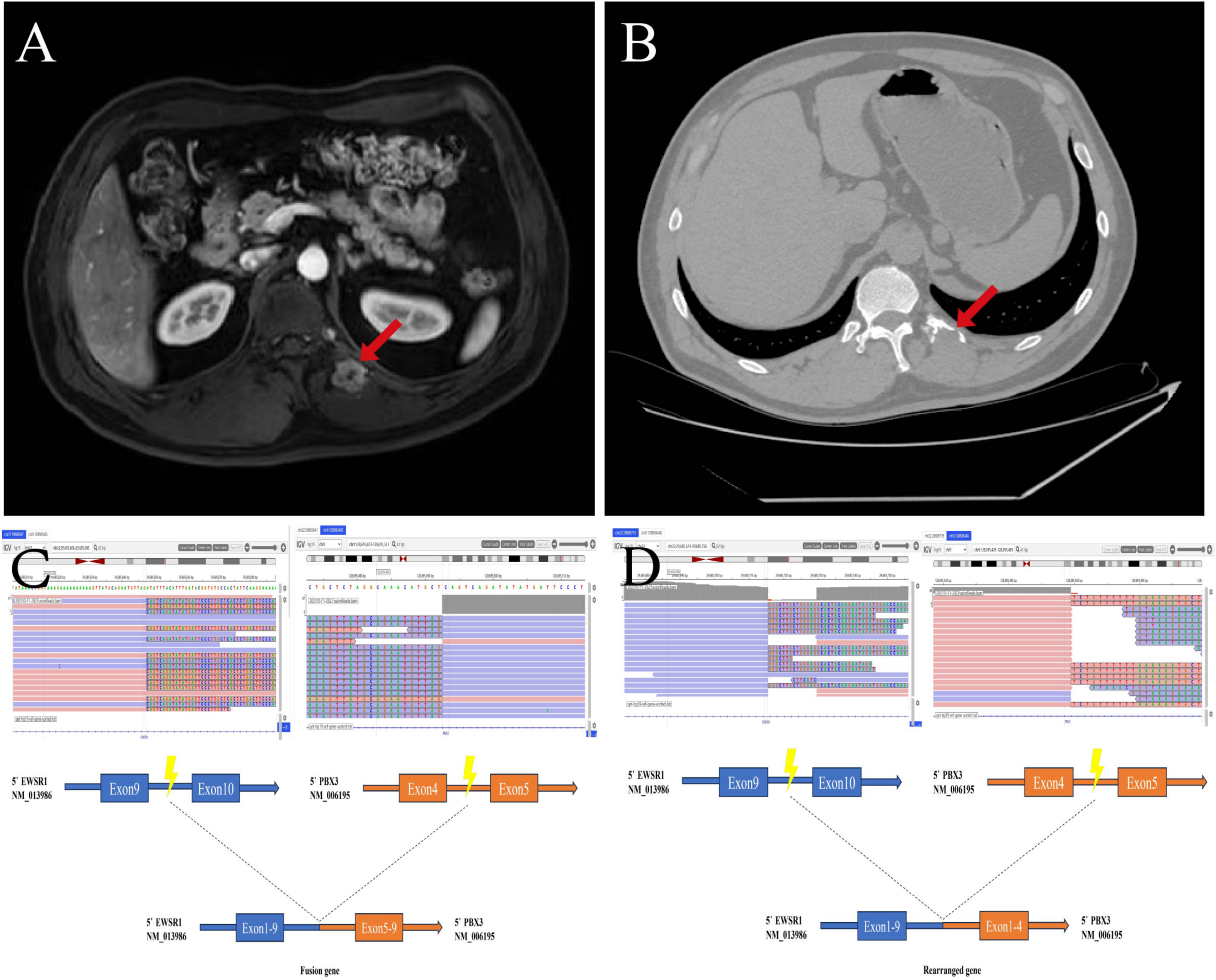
Imaging examination and molecular features. (A) MRI in axial plane, (B) Computed tomography in axial plane, (C) The fusion site was at exon 9 of the EWSR1 and exon 5 of the PBX3 gene. (D) The rearrangement site was at exon 9 of the EWSR1 and exon 4 of the PBX3 gene.
